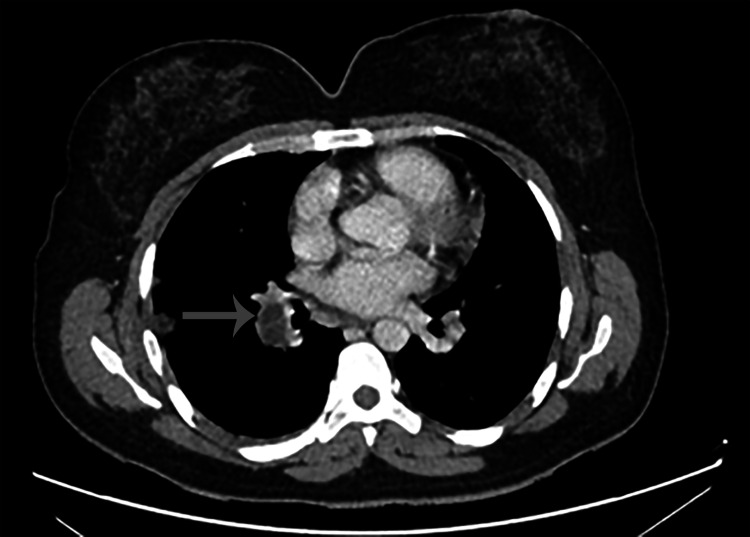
One-year follow-up CT scan.
